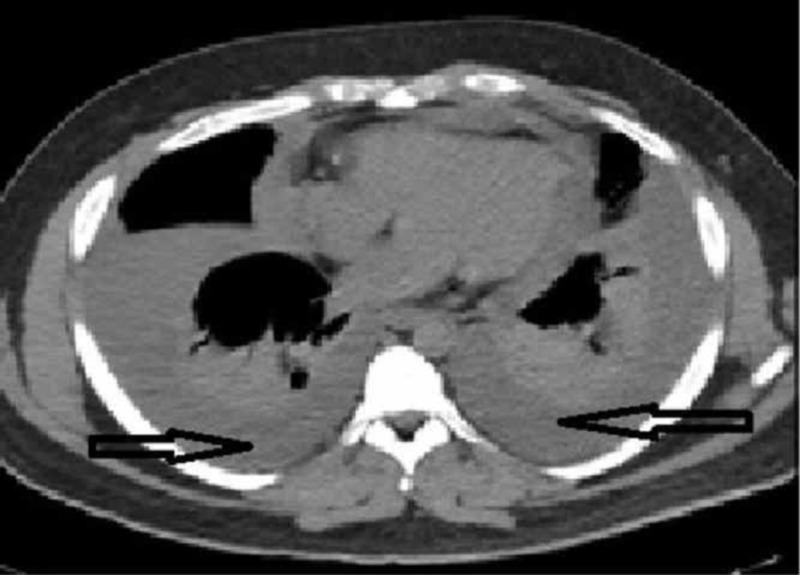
Computed tomography of chest (mediastinal window) showing pericardial effusion and bilateral pleural effusion Arrows pointing pleural and pericardial effusion
